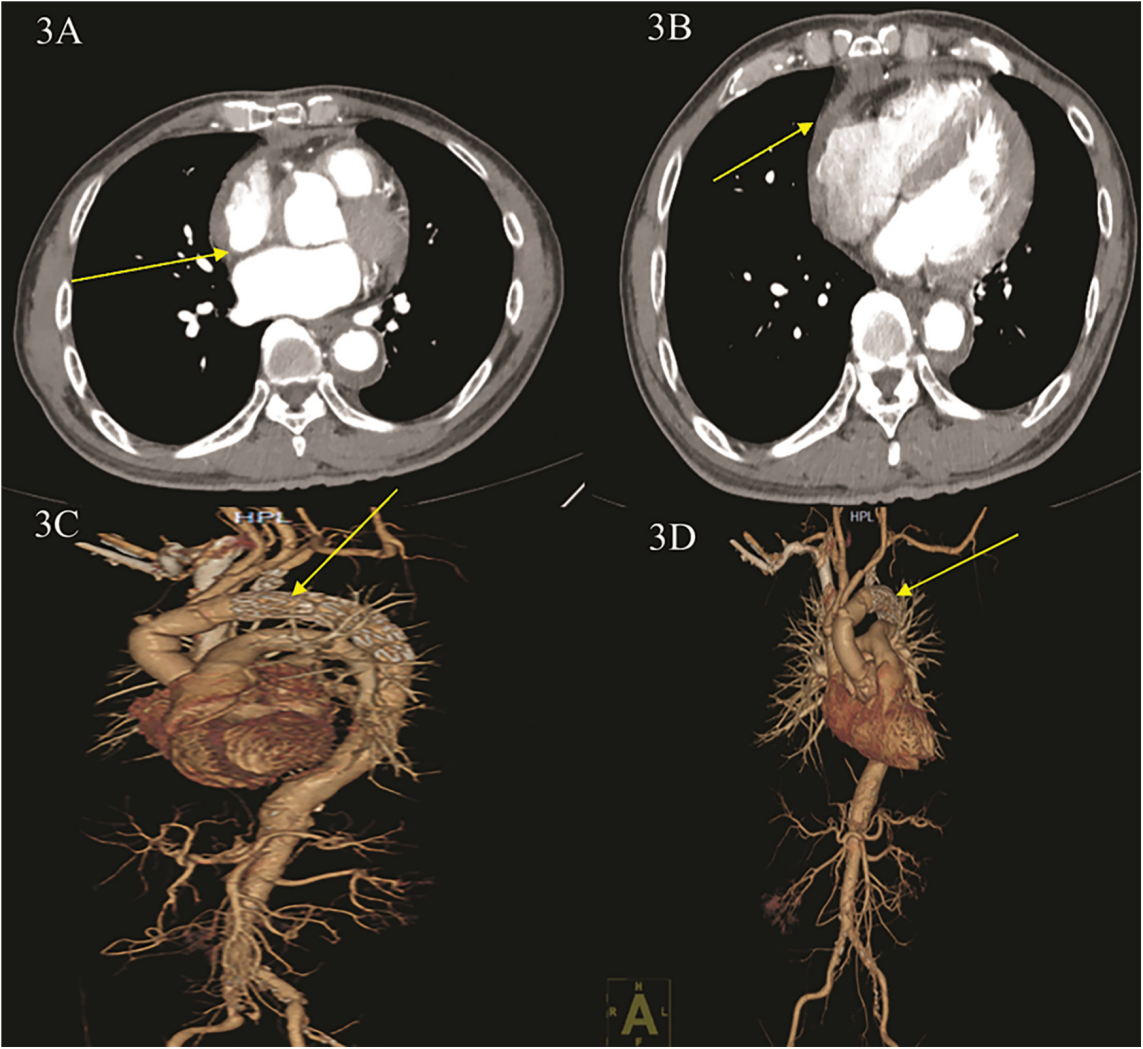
Postoperative CT angiography (CTA) of the thoracic aorta and abdominal and coronary arteries. (A,B) CTA shows: blood accumulation around the ascending aorta was significantly reduced, and right heart compression was reduced. (C,D) CTA three-dimensional reconstruction shows: ascending aortic replacement + total aortic arch replacement + stenting elephant nose after stenting, stenting position is good, no contrast leakage is seen.
